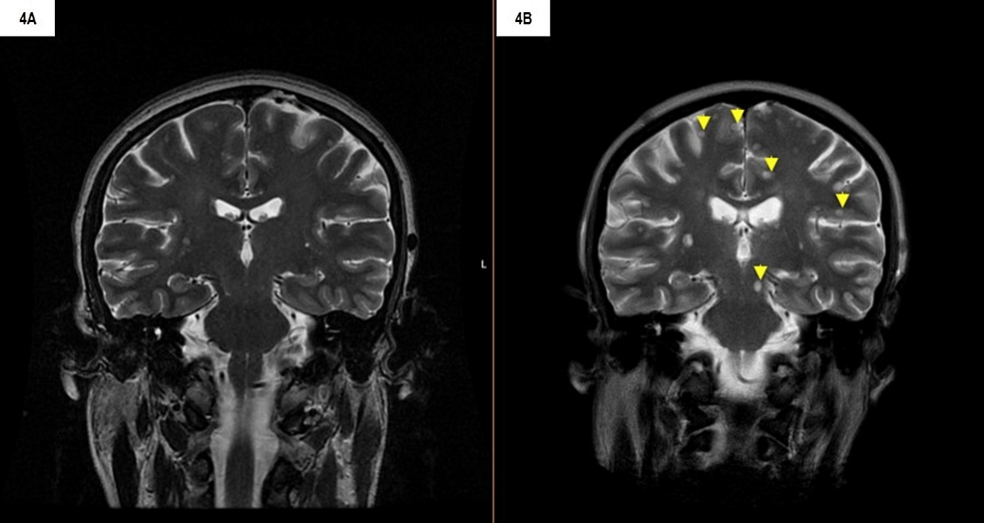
Cerebral magnetic resonance imaging showing cerebral disease progression—comparison between March 2020 (4A) and July 2021 (4B); yellow arrows: new cerebral lesions.
